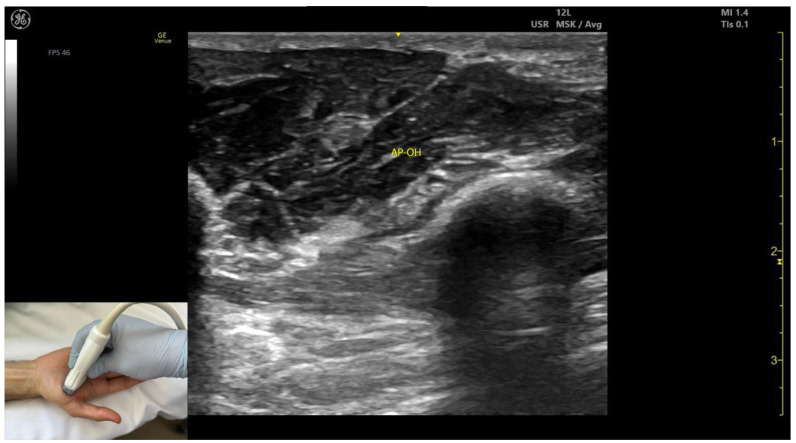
Ultrasound anatomy of the adductor pollicis oblique head (AP-OH) with key landmarks: AP-OH—adductor pollicis oblique head.
